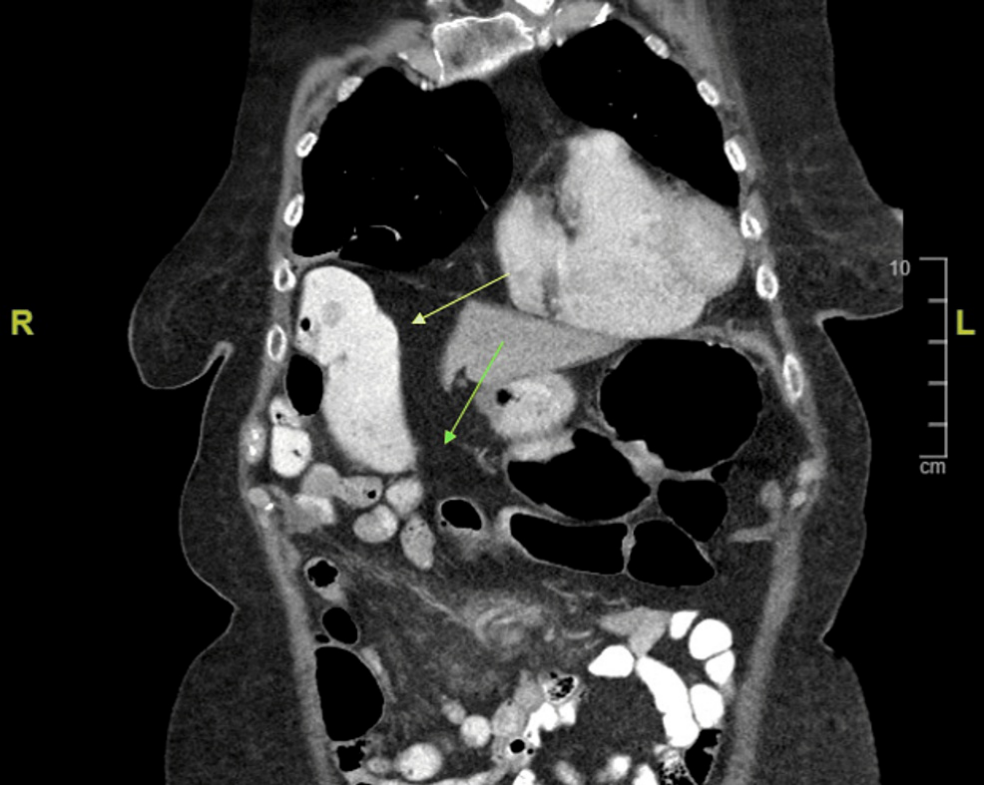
CT of the chest/abdomen/pelvis with contrast. The yellow arrow shows the initial portion of the transverse colon with contrast (opacified). The green arrow marks the mid portion of the transverse colon without contrast (not opacified) due to suspected obstruction.
